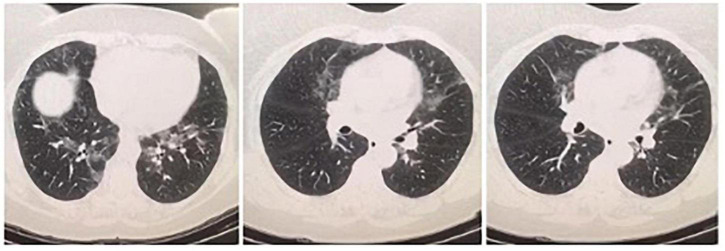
On her first HRCT result, bilateral central and peripheric ground glass opacities in upper and lower lung lobes and nodular thickening of right posterior pleura were noticed.
